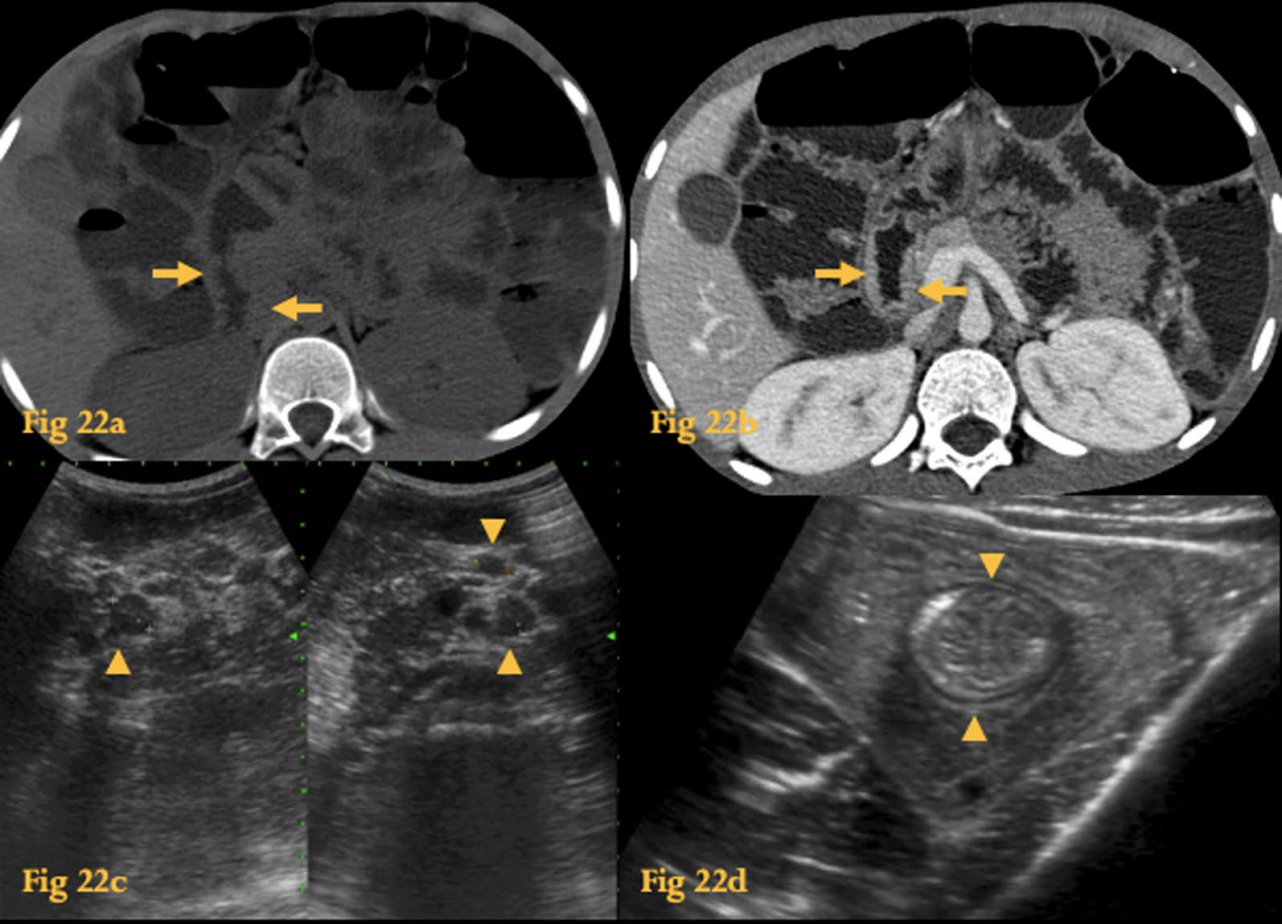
Celiac disease. Abdominal CT before (a) and after iv contrast administration (b) of a 14-year-old boy shows thickening and irregularity of the duodenal mucosa (arrows), with intense enhancement. Abdominal ultrasound of the same patient (c) detected several lymphadenopathies (arrowheads). Duodenal biopsy revealed disappearance of the “villi” associated with crypt hyperplasia, consistent with celiac disease. Abdominal ultrasound of another patient (d) who had three incidental transient small bowel intussusceptions (arrowhead), that were not present in a subsequent CT enterography a week later. Duodenal mucosa biopsy showed findings consistent with celiac disease
